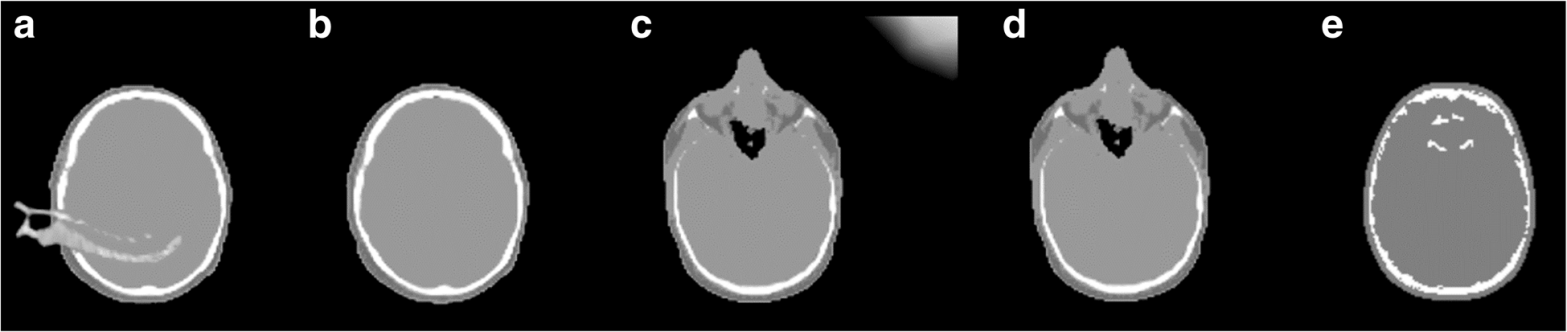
Typical bone artifacts found in attenuation maps from PET/MRI. a Dixon_Bone_ attenuation map with large infiltrative bone segment. Attenuation maps like this were only included in the study if the patient had a second acquisition yielding b an artifact-free attenuation map. c Dixon_Bone_ attenuation map with artifact in the upper right corner, which could be d manually removed. e UTE attenuation map with smaller bone segments inside the brain nearby the anterior ventricles. These were not removed
